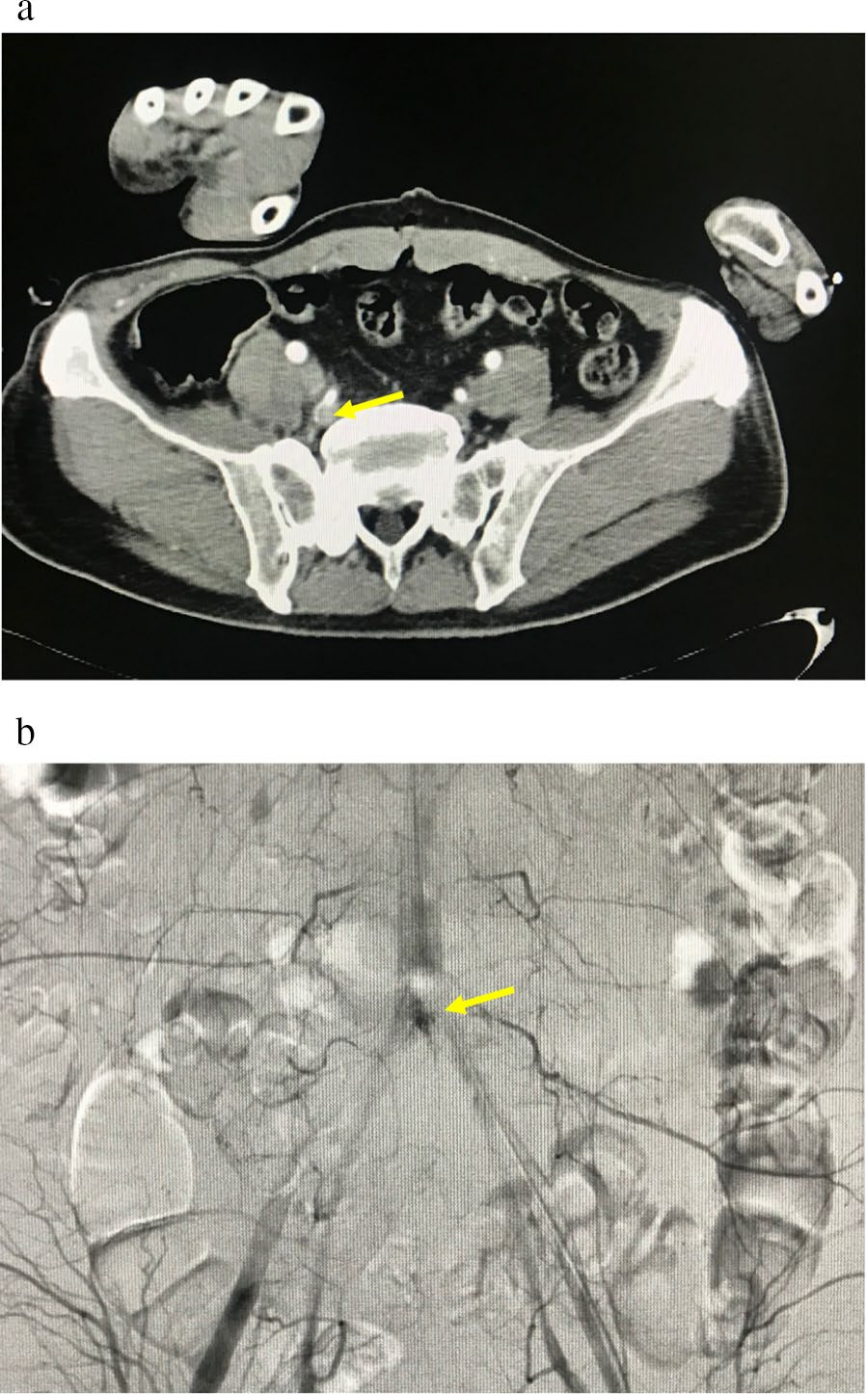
Contrast-enhanced computed tomography and X-ray images on admission. a Fractures of the 4th lumbar vertebra and right iliac body concomitant with arterial extravasation (yellow arrow) were detected. b We performed transcatheter arterial embolization to control this bleeding (yellow arrow)
